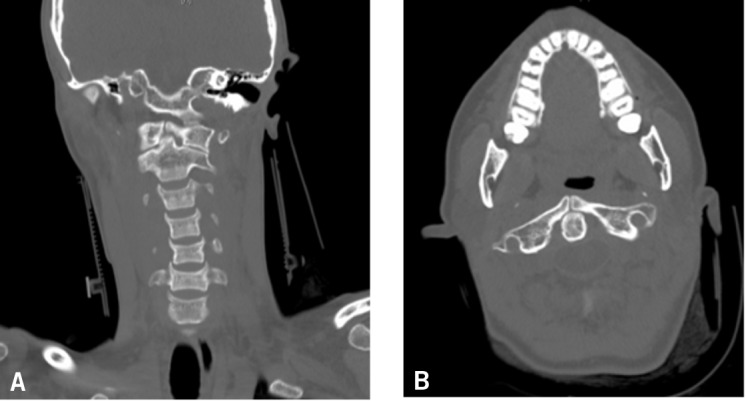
Coronal (A) and axial (B) computed tomography demonstrating non-fusion of the anterior arch of the atlas in the midline
